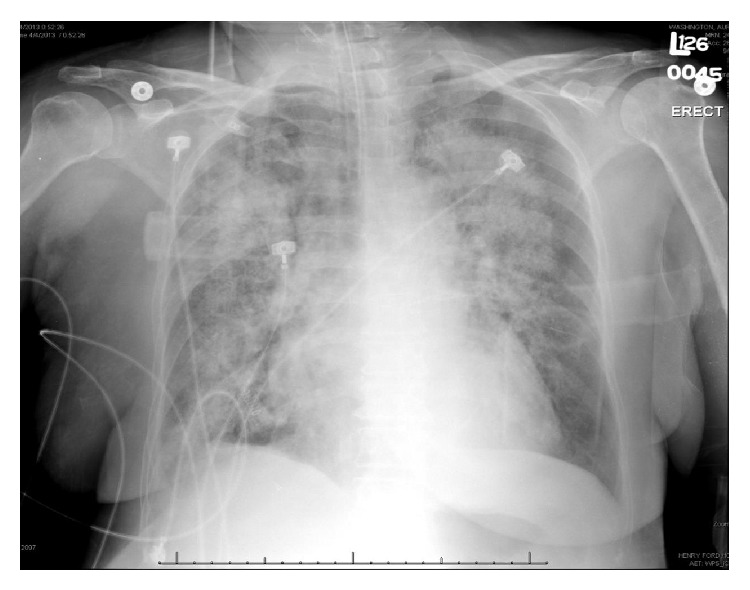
Chest X-ray revealing bilateral perihilar air space opacity. Tiny right pleural effusion with thickening or fluid within the fissures.
